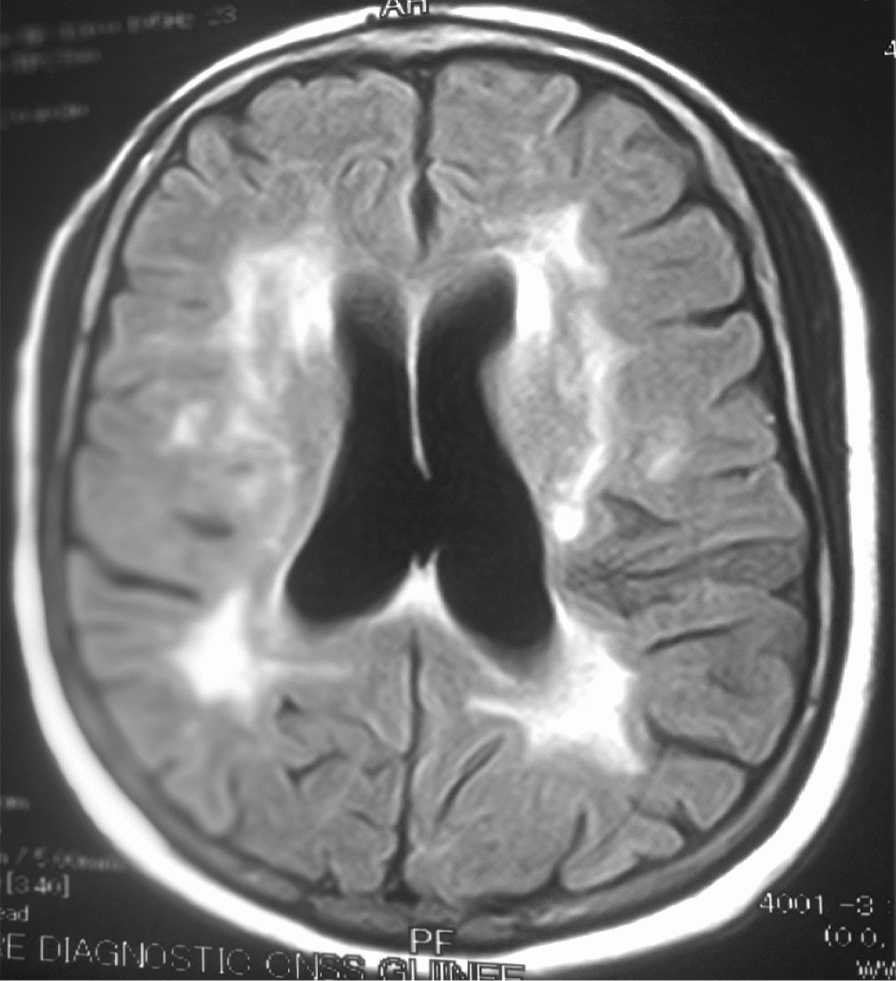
Cerebral MRI: focal abnormality in the subcortical white matter, consisting of a hypointense signal on the FLAIR-weighted image
